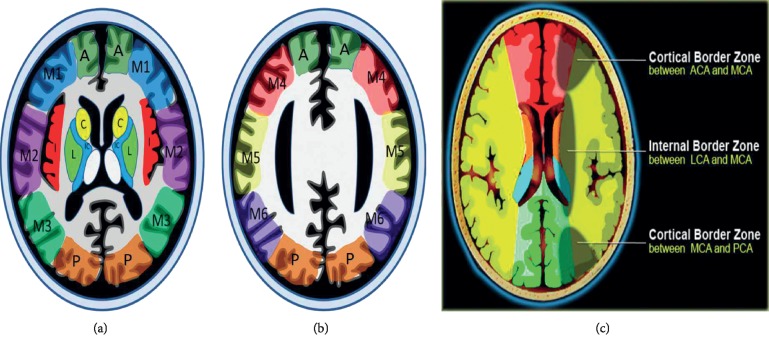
The Alberta Stroke Programme Early CT Score (ASPECTS) regions at the ganglionic (a) and supraganglionic (b) levels. The regions are the six cortical MCA regions (M1–M6), the insular cortex (I), the lentiform nuclei (L), the internal capsule (IC), the caudate head (C), ACA region (A), PCA region (P), and the three types of border zones (c).
